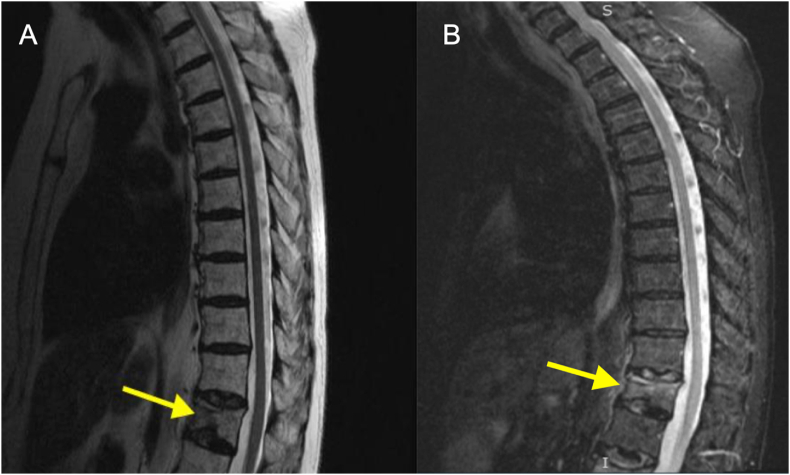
T12 vertebral compression fracture visualized using MRI sequences. (A) T2-weighted MRI with an arrow pointing to the fracture site. (B) STIR sequence with an arrow highlighting the bright signals indicative of edema/inflammation at the corresponding fracture location.
